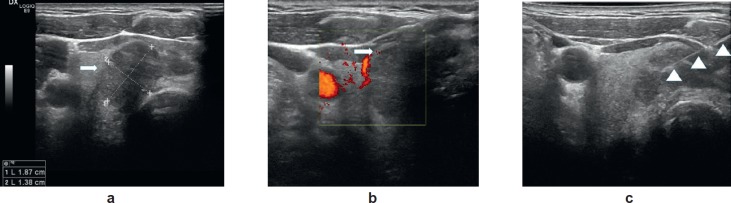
(a) Axial ultrasound image (6–13 MHz probe): a solid nodule measuring 19 × 14mm in the right thyroid lobe characterised by an incomplete peripheral hypoechoic halo and iso-, hypo-echogenic echotexture. (b) Axial ultrasound image with power Doppler: the absence of internal vascularity within the nodule (white arrow). (c) Fine-needle aspiration of the nodule (FNA); the needle (arrowheads) can be seen crossing the target.
